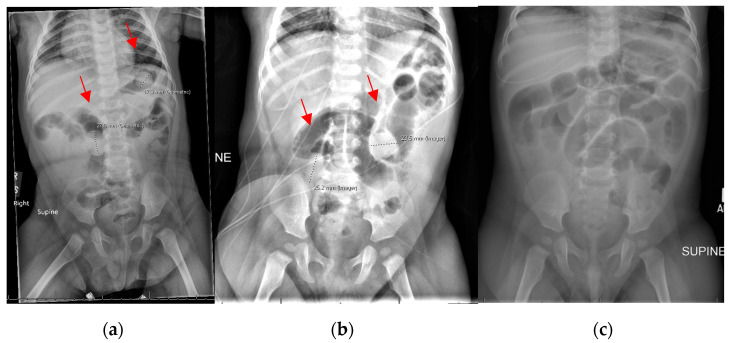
Serial abdominal X-rays obtained in an eight-month-old patient on hospital day one (a,b) and two (c) after admission for water bead ingestion. Arrows indicate expanded beads.
